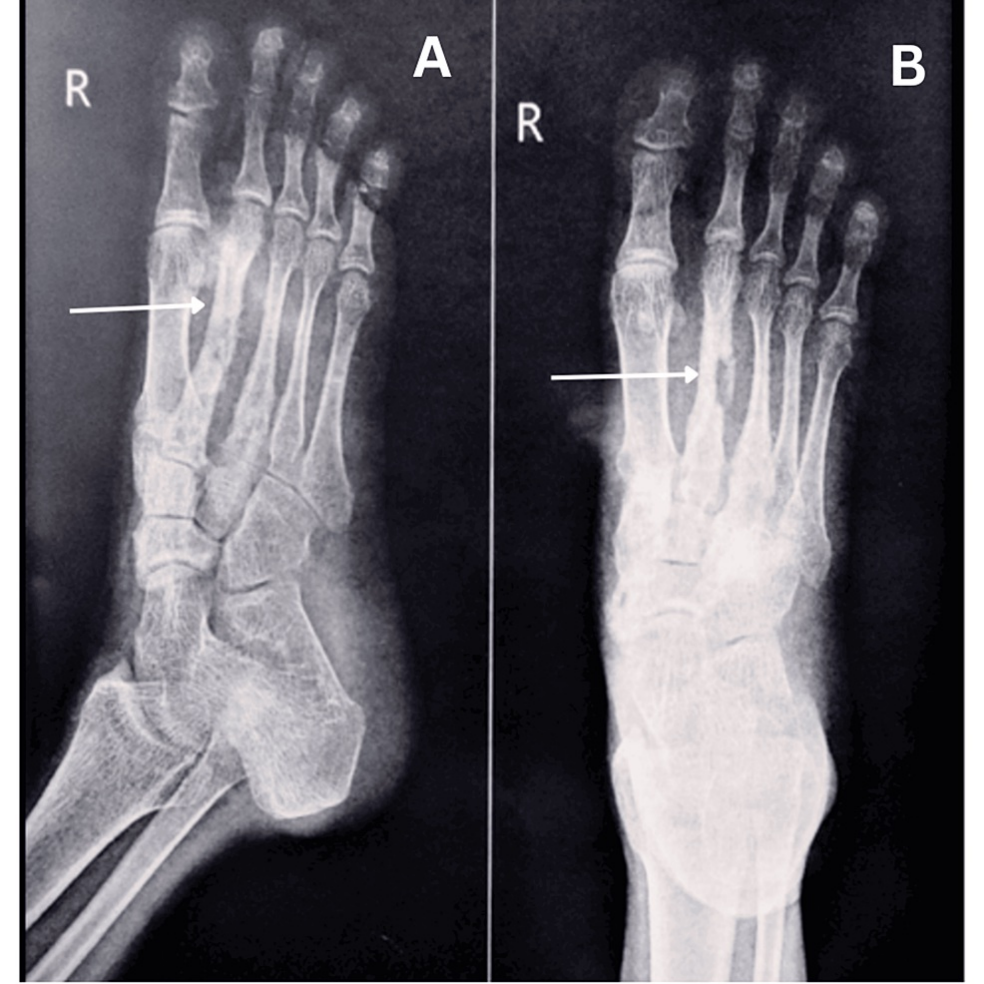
X-rays of the right foot after debridement and curettage of the second metatarsal: A) ankle lateral view and B) ankle AP view AP: anteroposterior
